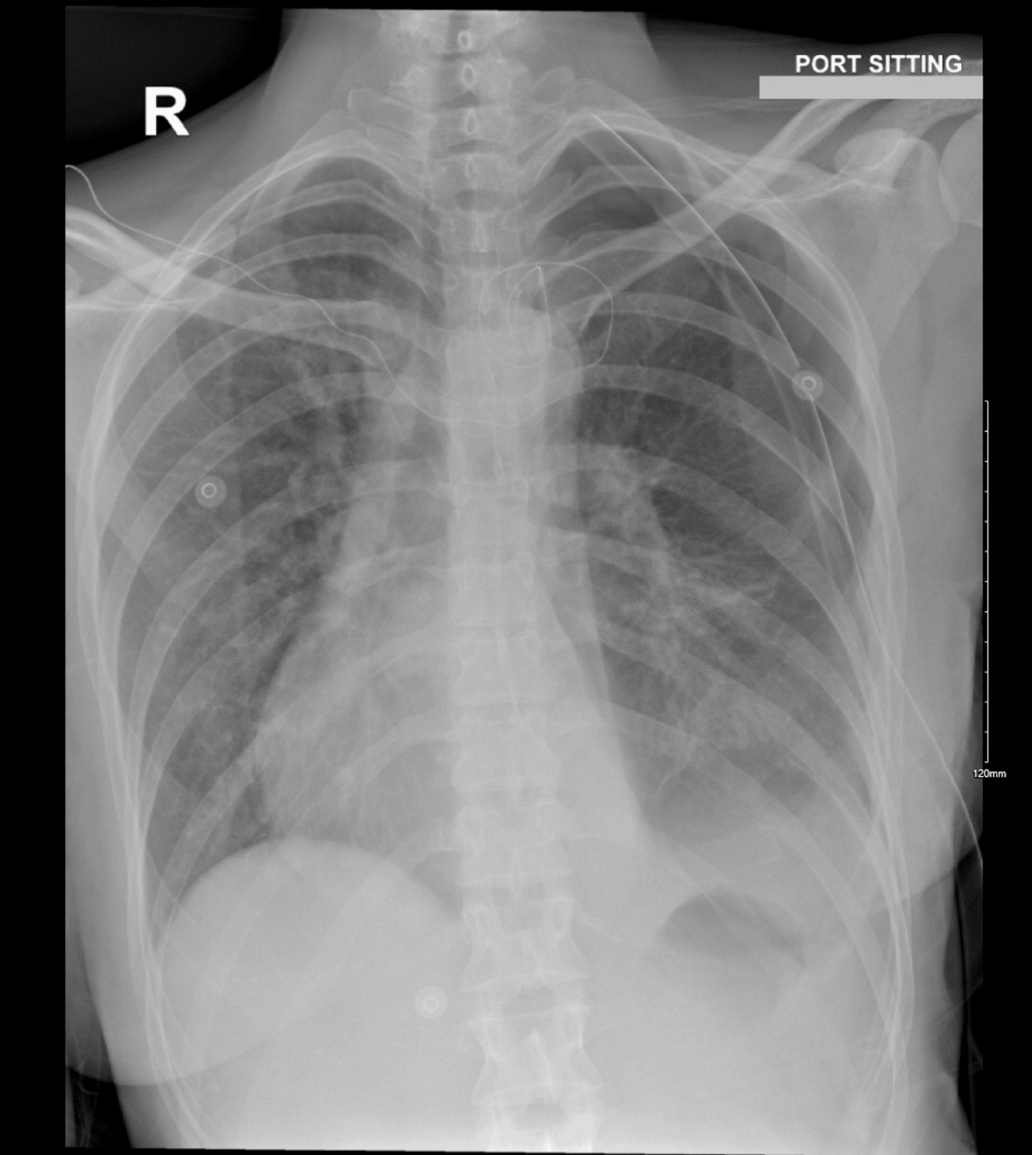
Postoperative chest X-ray demonstrating satisfactory expansion of the left lung
